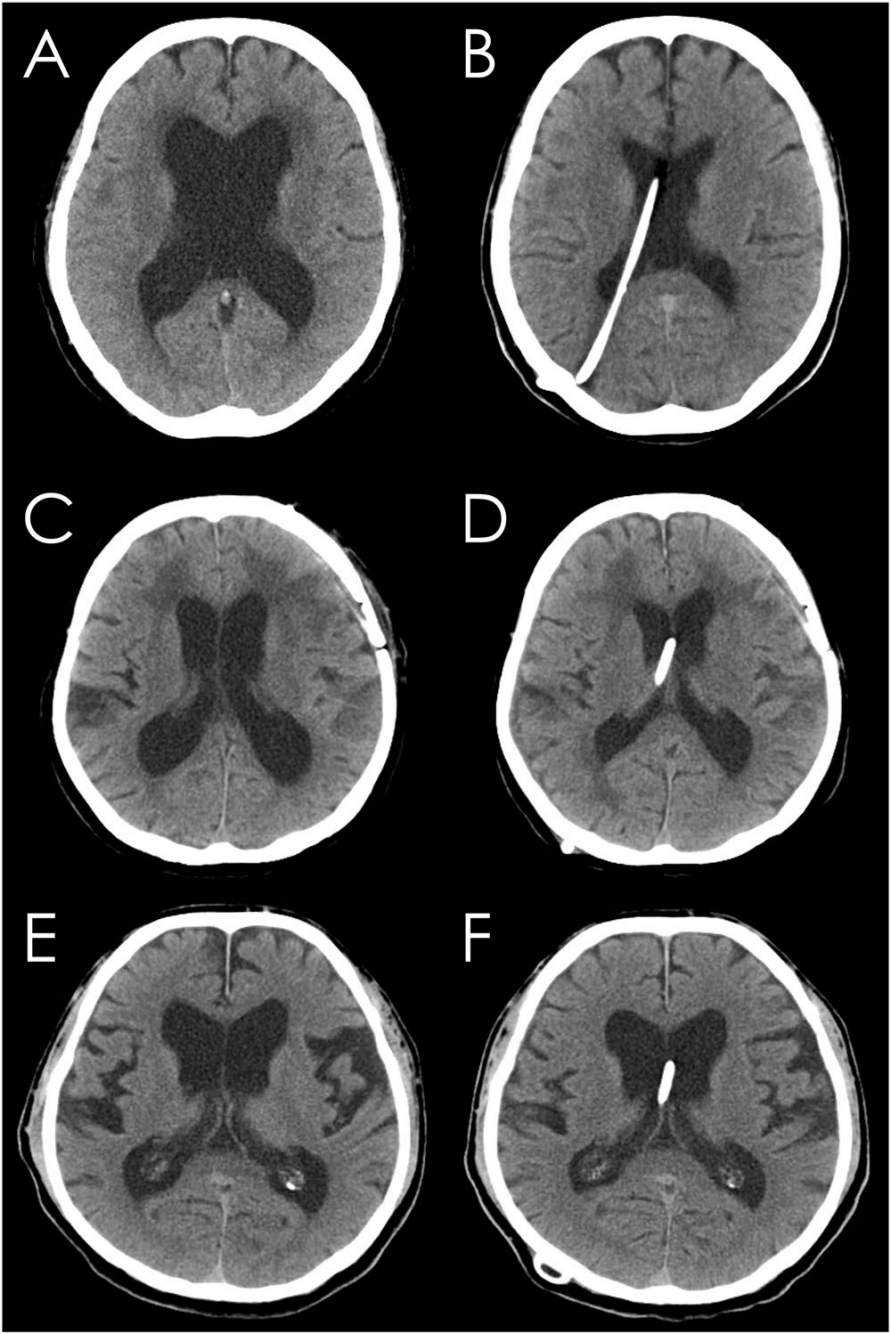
Conventional CT scan one day before and one month after shunt surgery. Representative cases diagnosed with adult-onset congenital NPH (A,B), secondary NPH (C,D) and idiopathic NPH (E,F).
